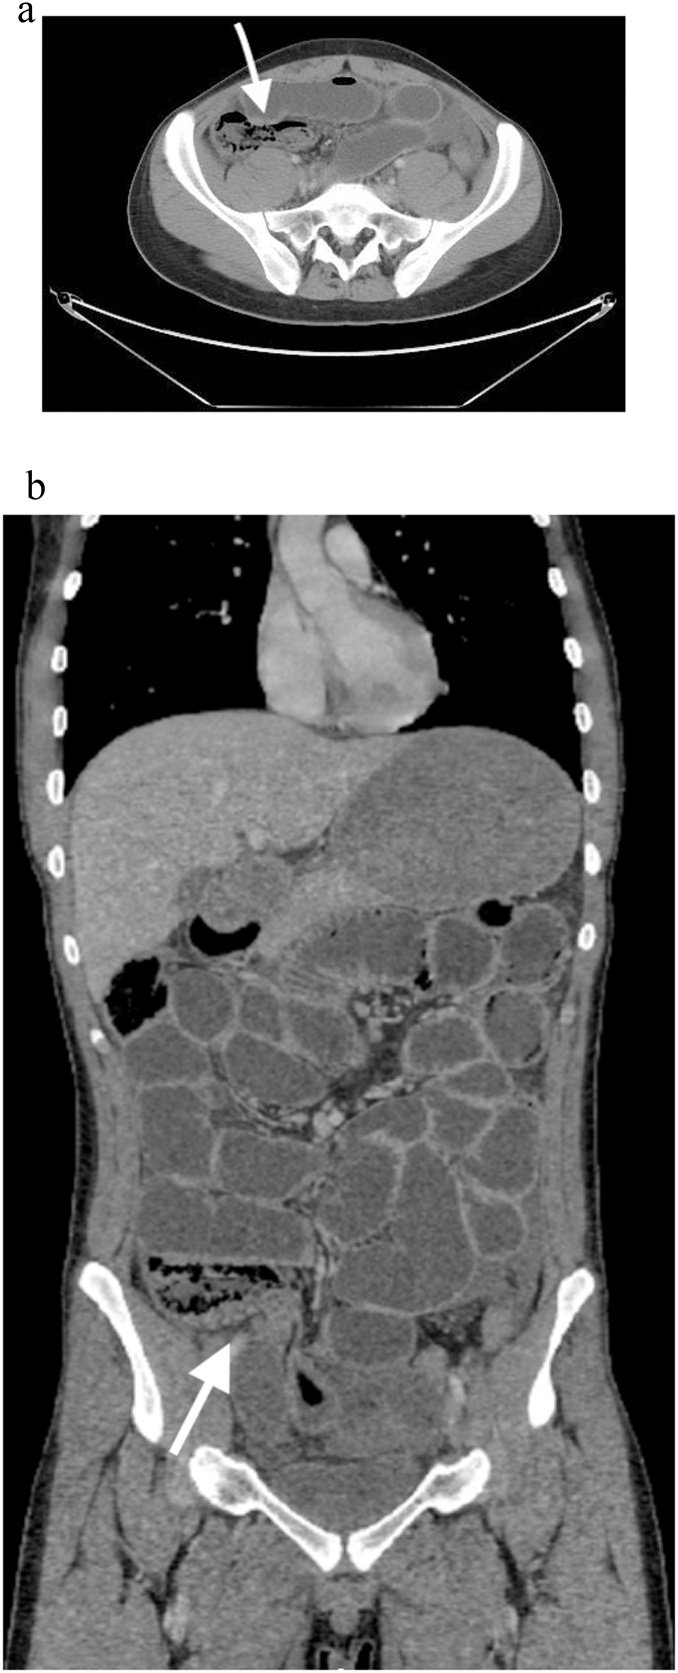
A and B: CT scan of the abdomen demonstrating dilated small bowel, transitional zone of the obstruction, and evidence of pneumatosis intestinalis.
